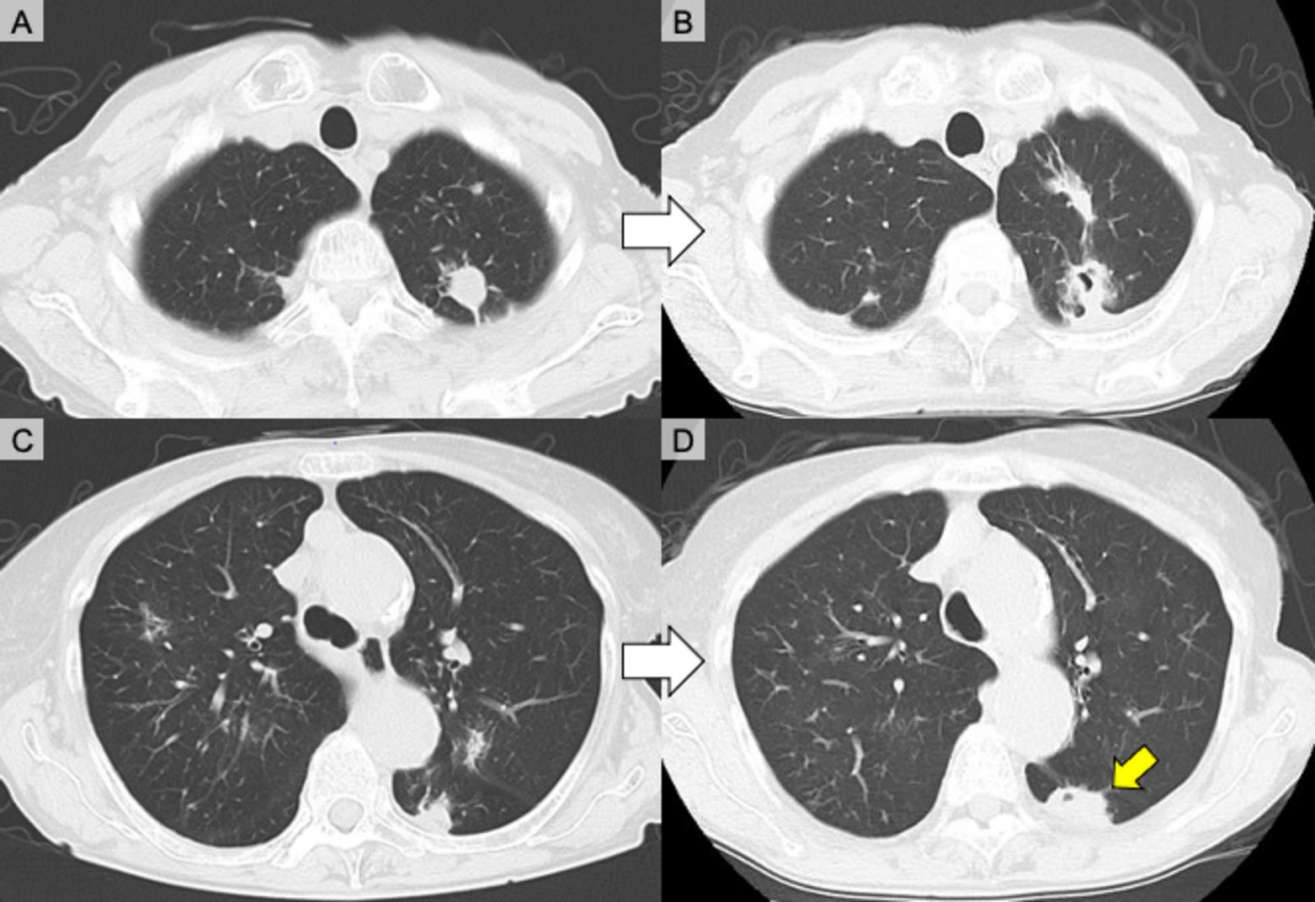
Chest computed tomography (CT) images of serial pulmonary lesion changes before treatment. (A, B) Axial CT images of the same slice obtained approximately 1 year apart showing progression of a left upper lobe nodule with cavitation development. (C, D) Axial CT images of another slice demonstrating progressive enlargement of nodular consolidations in both lungs with new cavitation over the course of 1 year. (D) During video‐assisted thoracoscopic surgery, a biopsy sample was obtained from a lesion in the area indicated by the yellow arrow.
